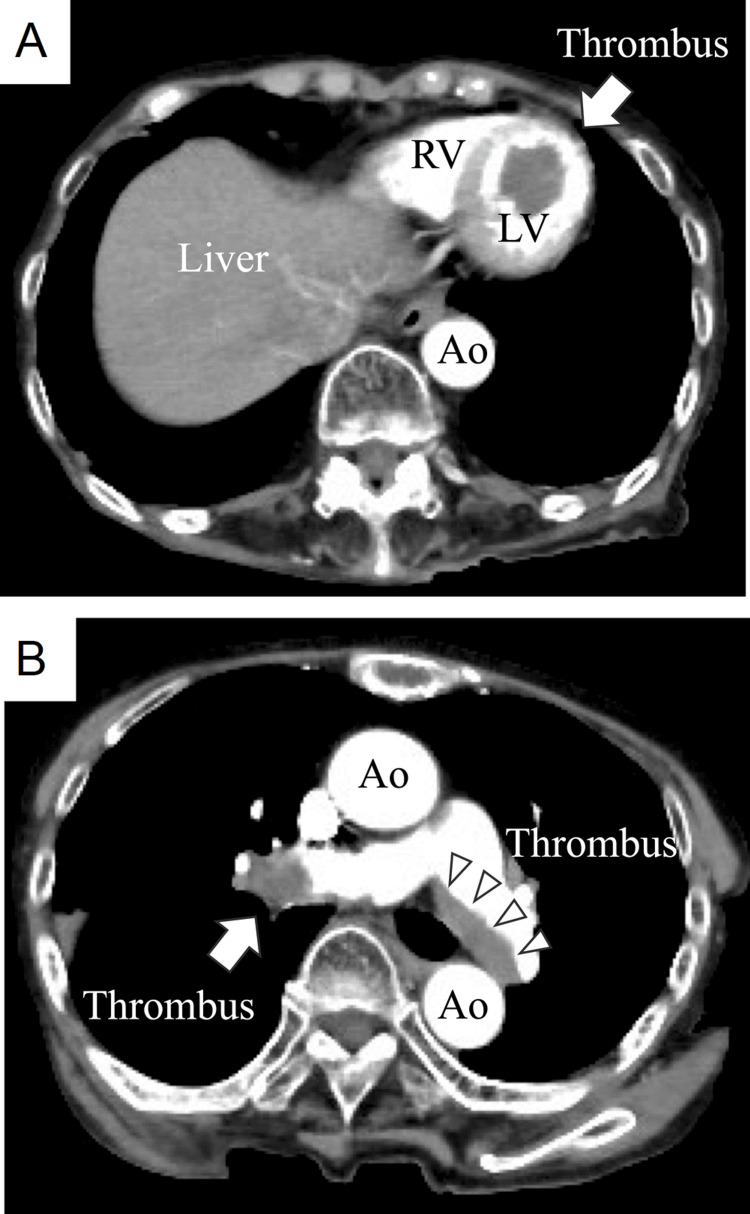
CT shows (A) a giant 30×18 mm thrombus in the left ventricular apex (white arrow) and (B) A new large thrombus in the right pulmonary artery (white arrow) and a mural thrombus in the left pulmonary artery (white arrowheads) Ao, aorta; LV, left ventricle; RV, right ventricle
